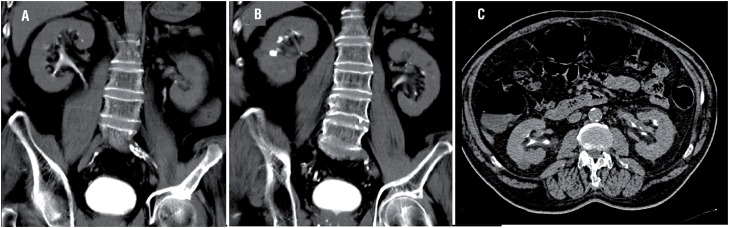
(A, B) Coronal and (C) axial scans of computed tomography (CT) urography without signs of UUT disease.
